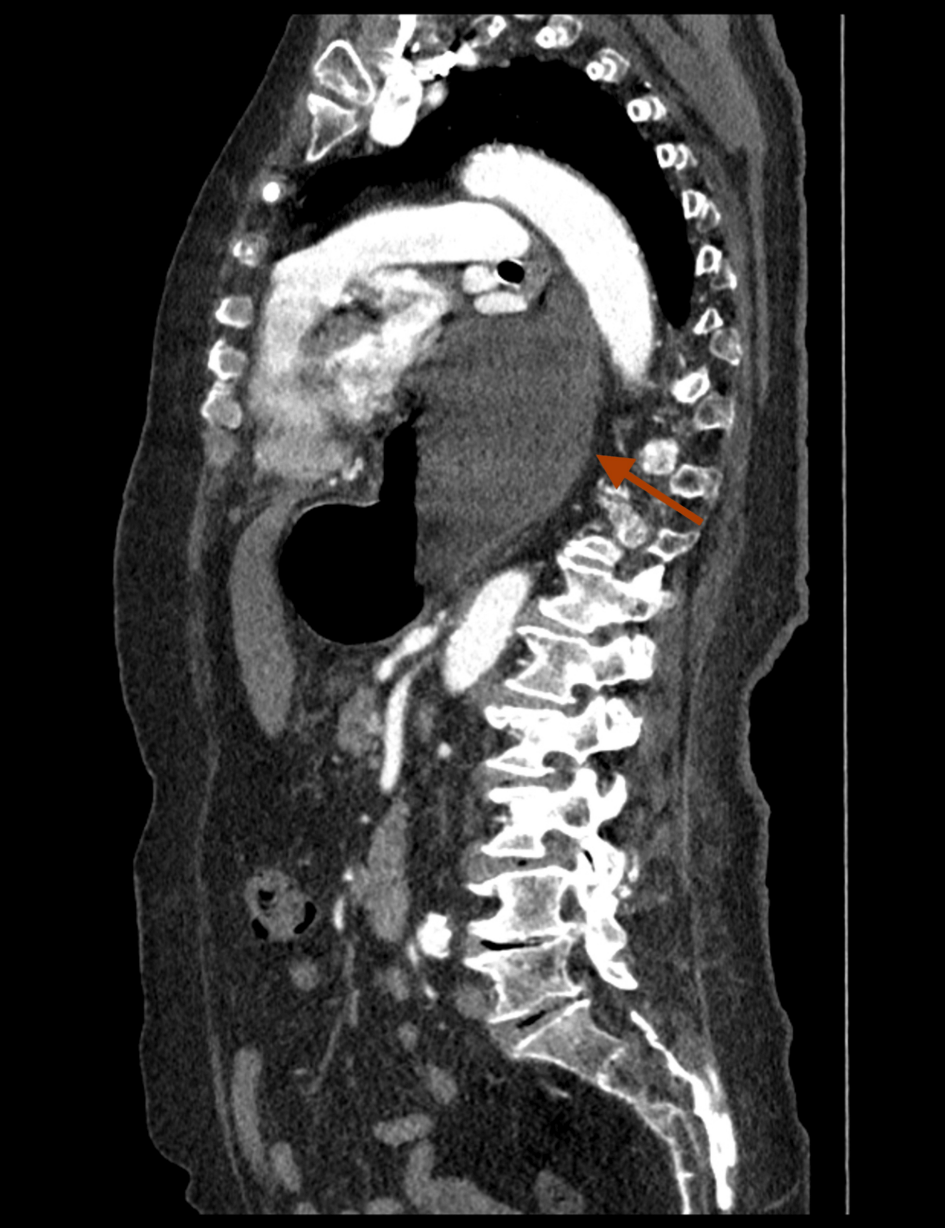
Chest CT showing incarcerated sliding hiatal hernia (sagittal). The red arrow indicates the hernia, visible as a retrocardiac mass. The presence of an air-fluid level within the mass supports the diagnosis.
